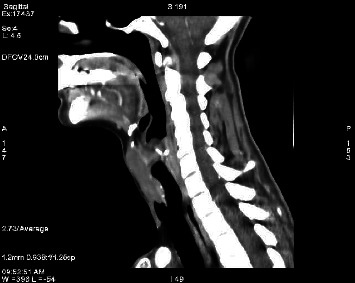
CT scan 6 months post-op showing successful reduction in goiter size through medical treatment.
